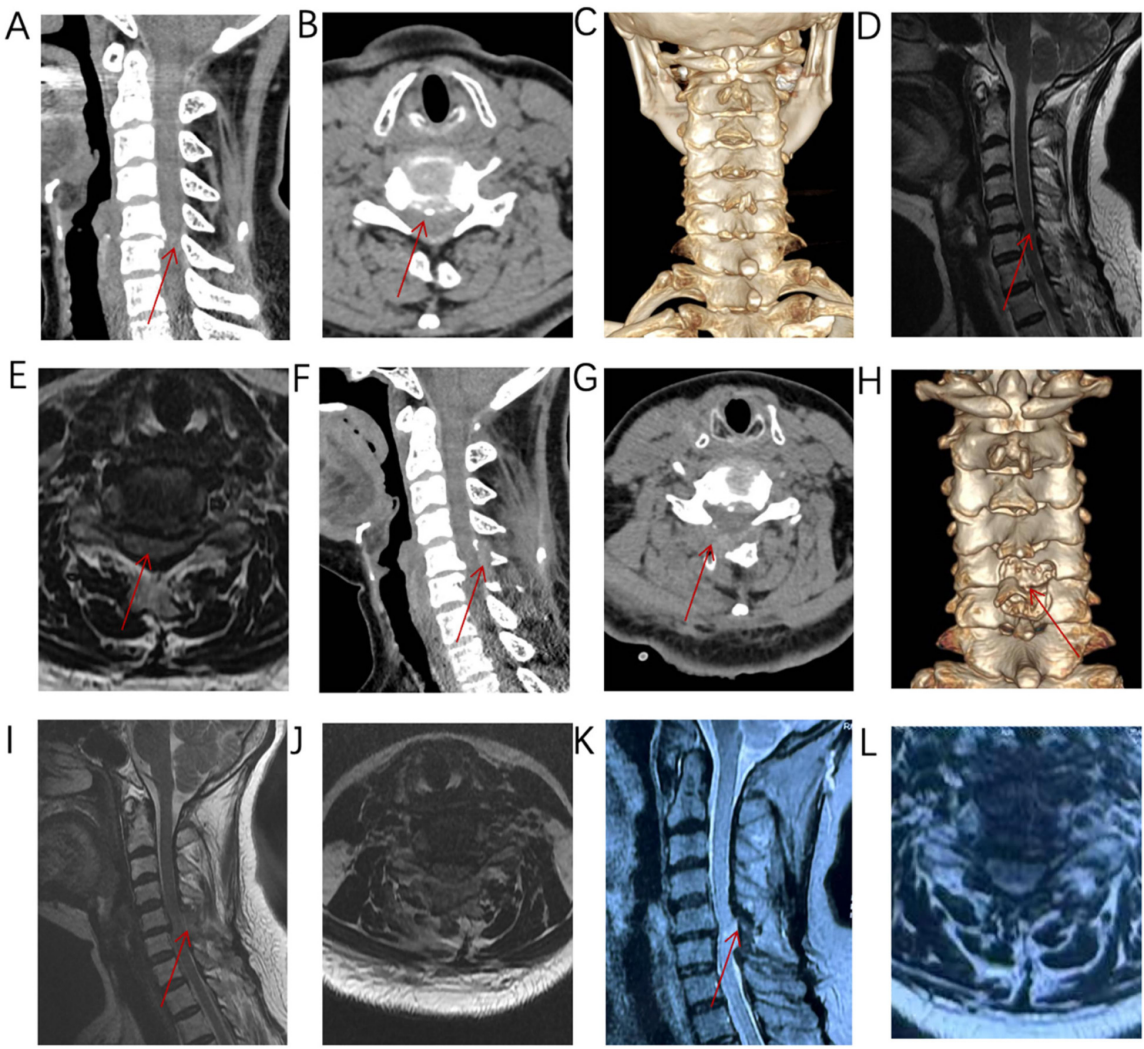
(A–E) Imaging reveals anterior and posterior compression at C5/6, consistent with the diagnosis of pinching cervical spondylotic myelopathy. (F–J) Postoperative imaging shows adequate decompression, with the inferior lamina decompression range exceeding half of the lamina. (K–L) MRI imaging at 10 months after surgery shows that the spinal cord decompression is sufficient.
